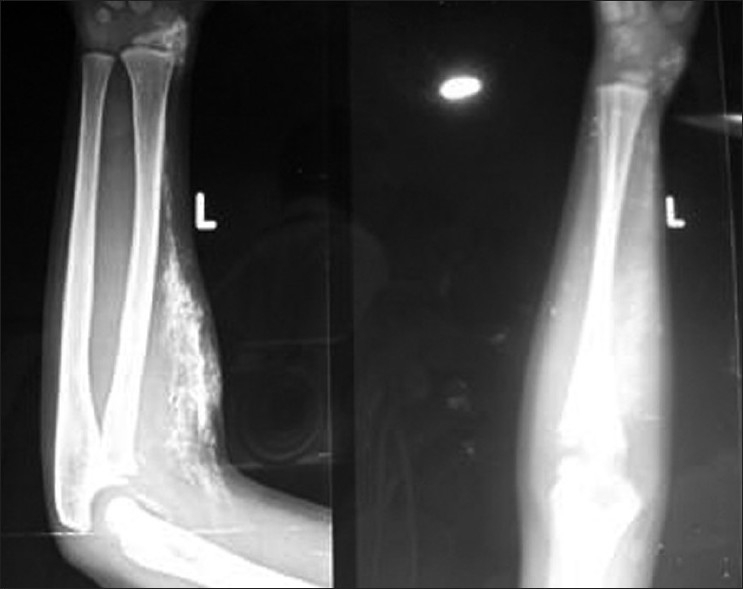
X-rays of forearm with elbow joint (anteroposterior and lateral views) showing ossification in the dermis, subcutaneous tissue, and muscles
